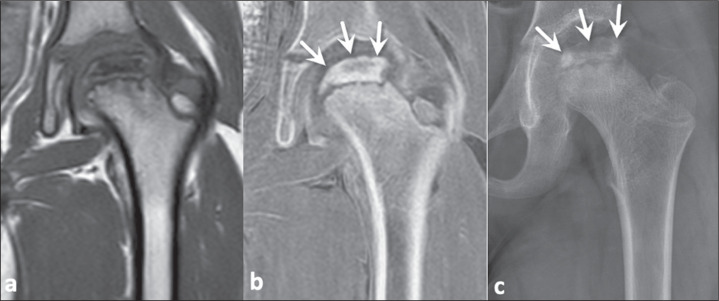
An 8-year-old boy with Legg-Calvé-Perthes disease. T1-weighted MRI
